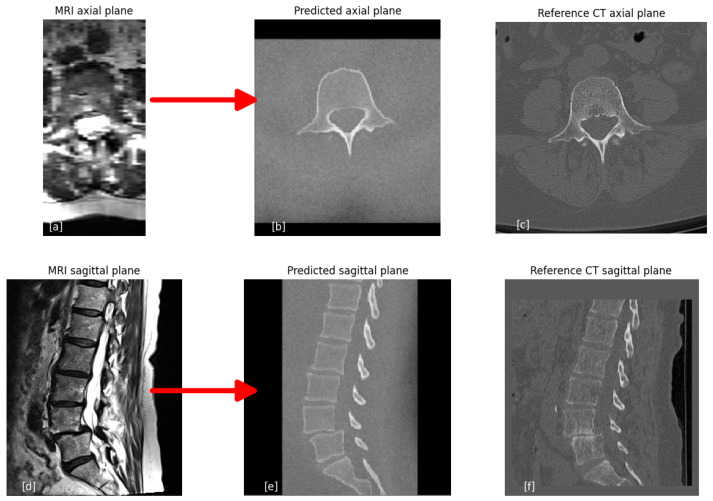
Conversion from MRI to sCT. Example slice from the input MRI T2 protocol of the (a) axial and (d) sagittal slices. The output sCT based on the input MRI is presented, viewing the (b) axial and (e) sagittal slices. Right: The reference CT of the same patient with similar (c) axial and (f) sagittal slices.
